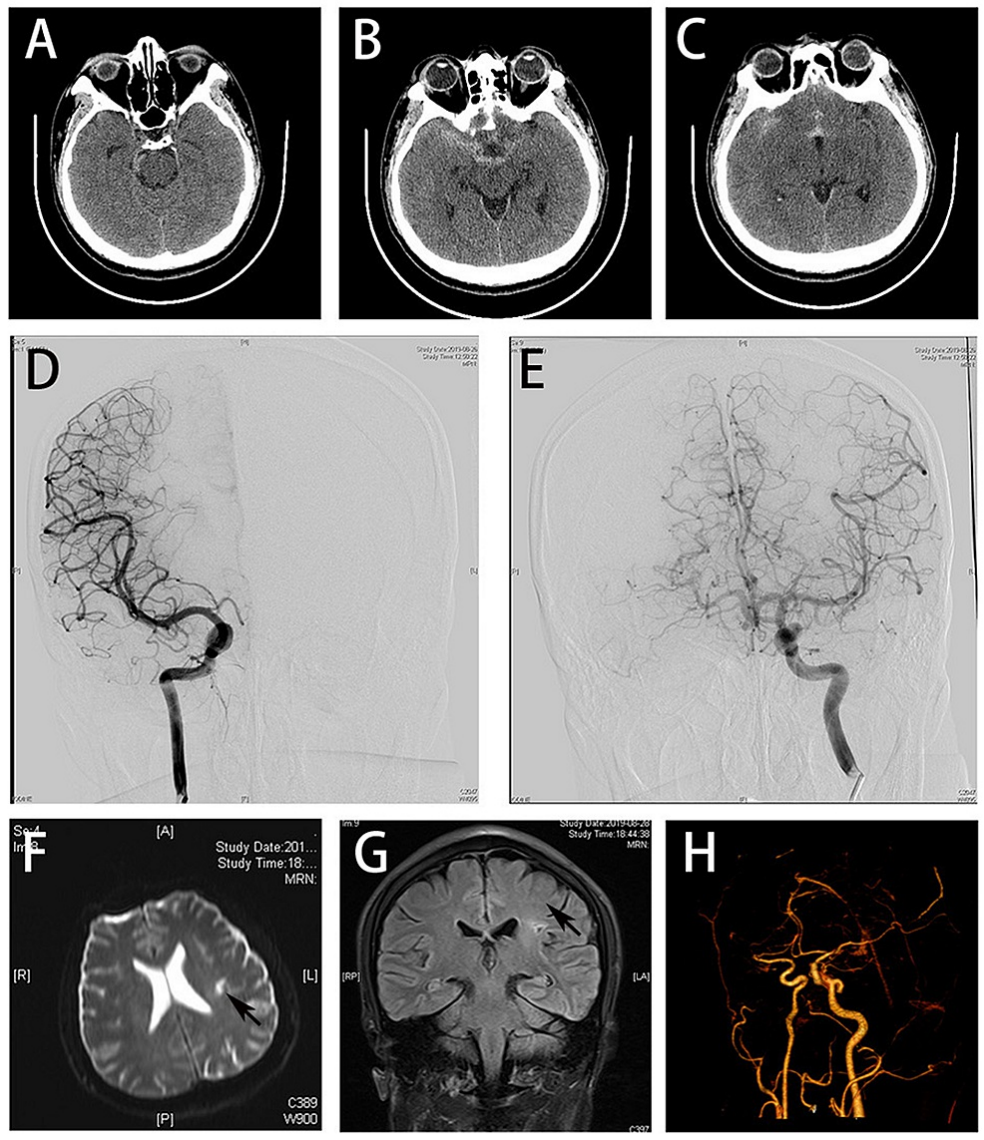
Multimodal neuroimaging of the patient (A-C) The emergent brain CT showed diffused SAH. (D and E) DSA on day two revealed no aneurysm. (F and G) Brain MRI showed previous stroke in left frontal lobe (black arrow). (H) Cerebral and cervical CTA performed at discharge revealed no aneurysm. SHA: subarachnoid hemorrhage; DSA: digital subtraction angiography; CTA: computed tomographic angiography
